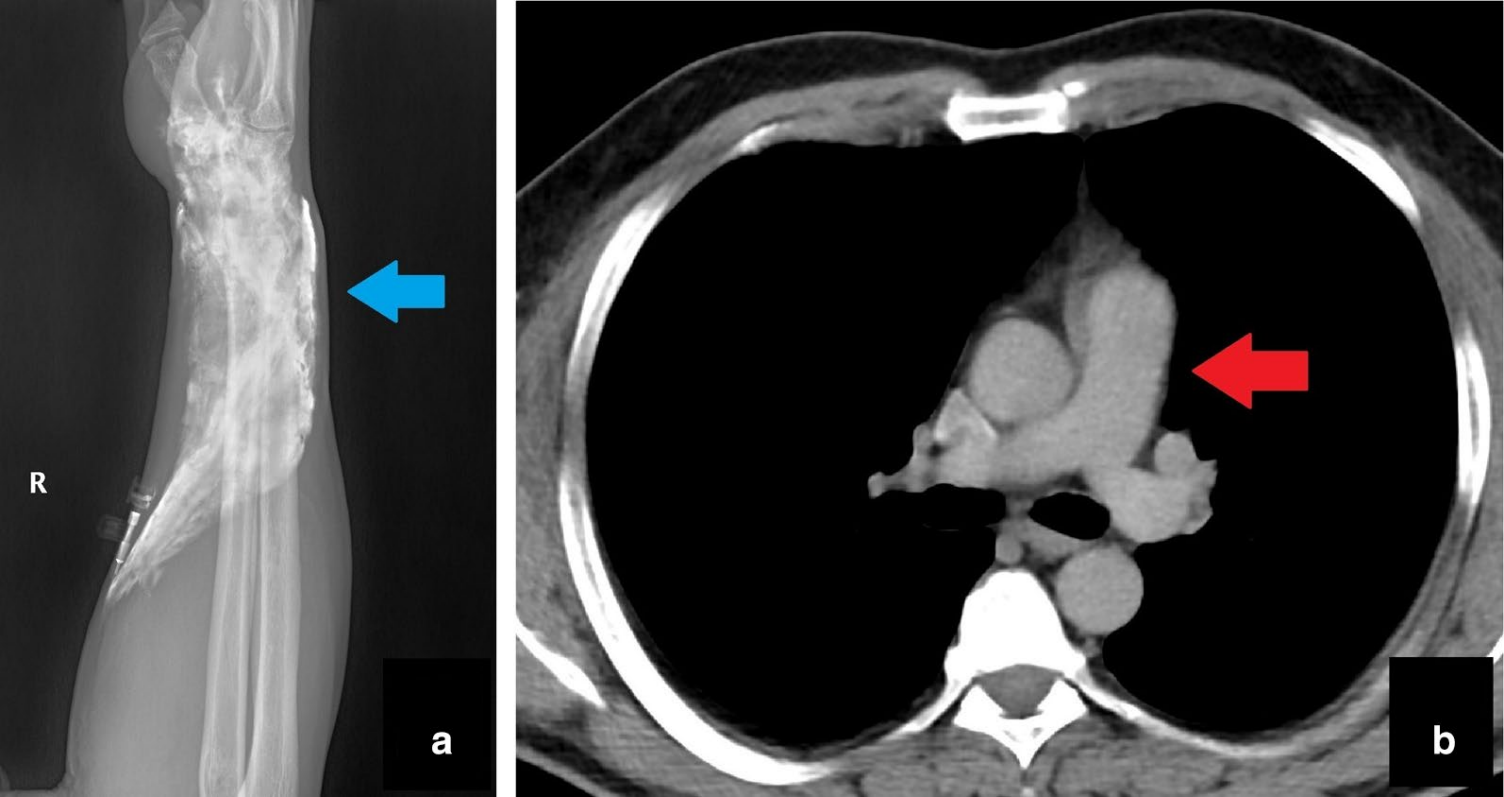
A complication (Type 11 error) causing acute harm to the patient and resulting in an insufficient examination. Thorax CT with pulmonary thromboembolism (PTE) protocol was performed for a dyspneic patient with a known breast cancer history. Just after the study, the patient complained of pain and swelling in the forearm. The X-ray of the upper extremity showed extravasated contrast agent to the wrist region (blue arrow, a). It was noted that the amount of intravascular contrast media was not sufficient for the evaluation of PTE (red arrow, b). Medical treatment was given, and the procedure was repeated. This case is a good example of “acute and temporary harm” caused by a periprocedural complication
